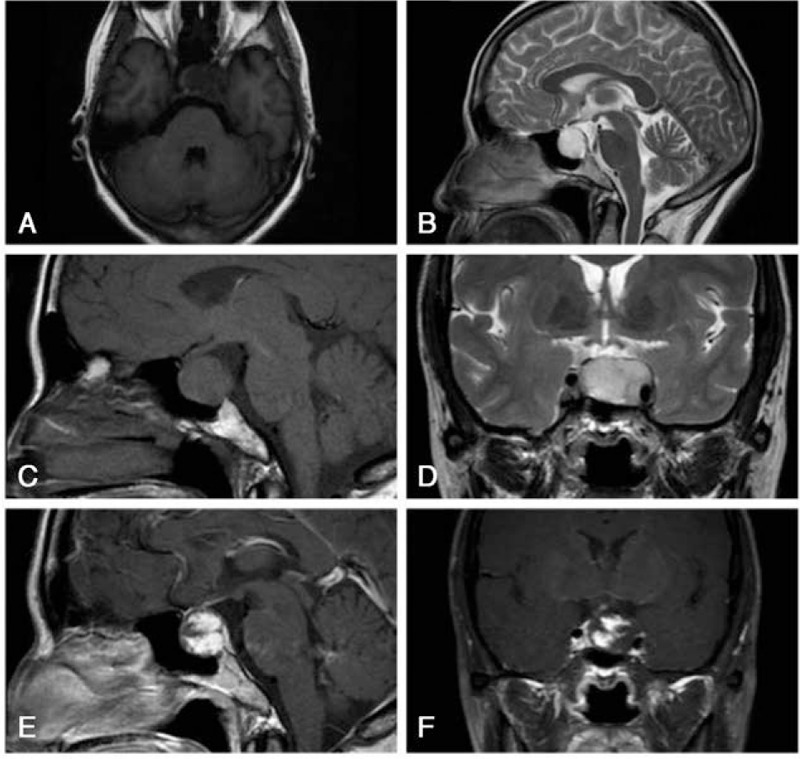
Magnetic resonance image showing a mass lesion in the sellar region with a size of 2.7 cm × 1.7 cm and a clear boundary. The lesion is located mostly in the intrasellar region but had pushed the optic chiasm slightly upward and invaded the left cavernous sinus. It appeared isointense on T1-weighted images (a and c) and hyperintense on T2-weighted images (b and d). Gadolinium contrast revealing heterogeneous enhancement with a mulberry-like appearance after gadolinium injection (e and f).
